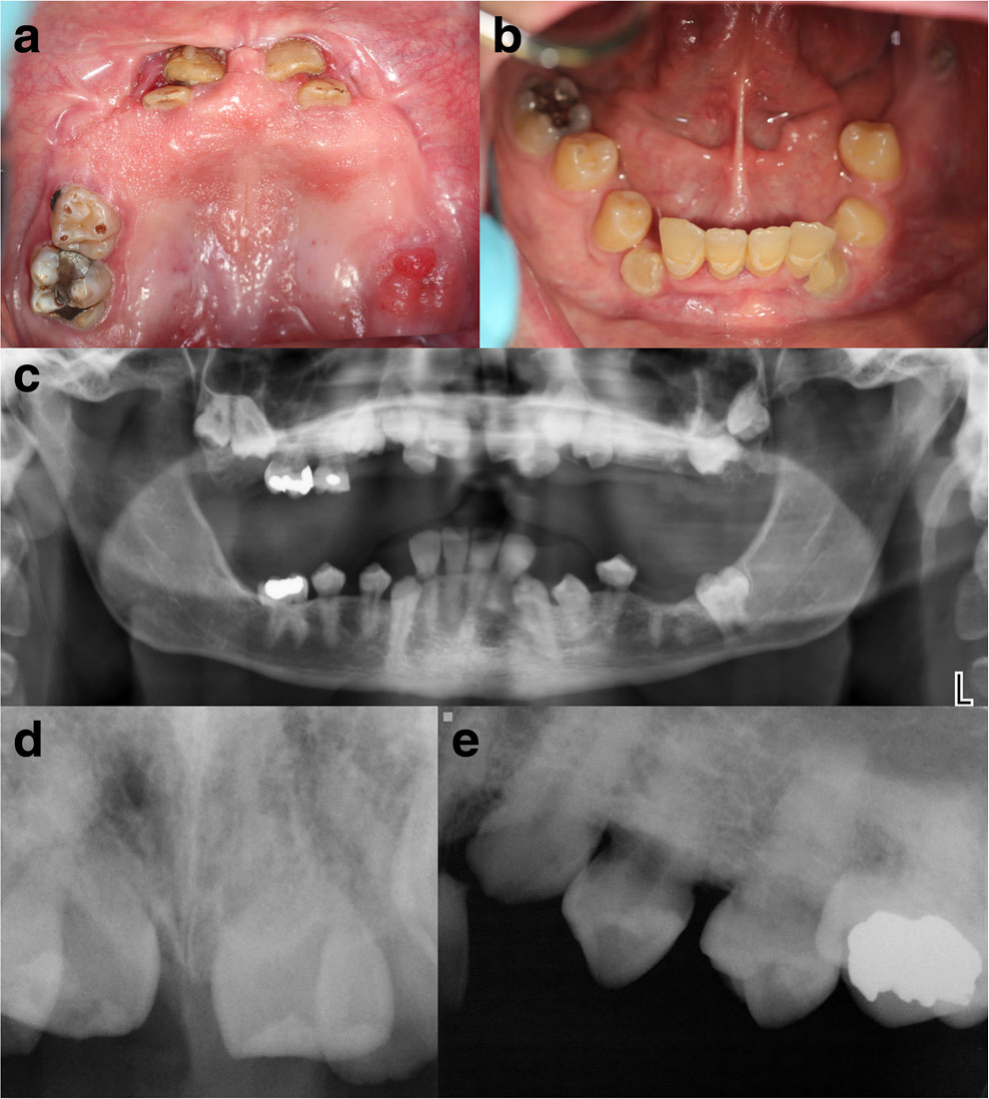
Dental manifestations of SMS. Clinical photography of maxillary a and mandibular b teeth showing failure of eruption of many permanent teeth. A dental panoramic radiograph c confirmed that most of these permanent teeth were impacted in the bone. d Anterior periapical radiograph showing very short roots. e Periapical view of upper left quadrant teeth revealing absent periodontal ligament space consistent with ankylosis
